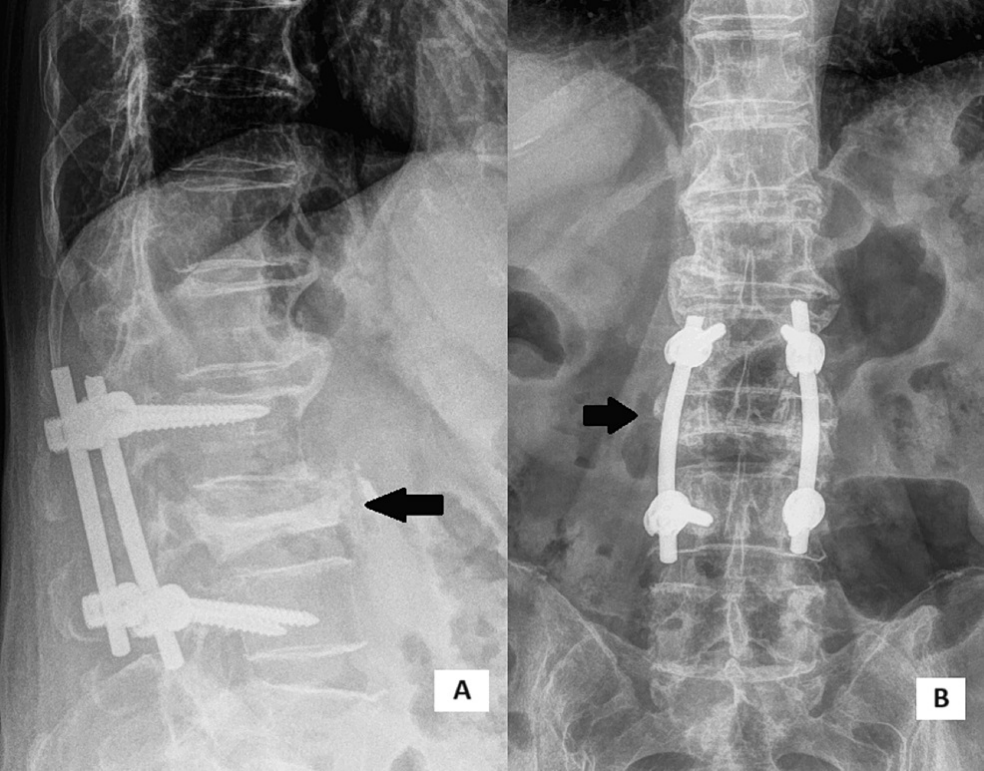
Postoperative radiographs of posterolateral instrumented fusion (PLF) (L2 to L4). The black arrow presents the OVF of L3. Lateral (A) and anteroposterior (B) views.
